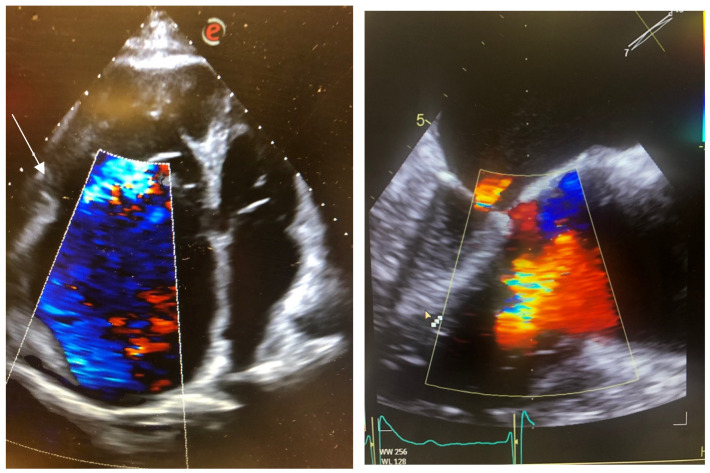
Apical 4-chamber TTE (left) and mid esophageal TEE (right) of Ebstein’s disease with giant right atrium and low tricuspid insertion (withe arrow) with a wide coaptation gap.
